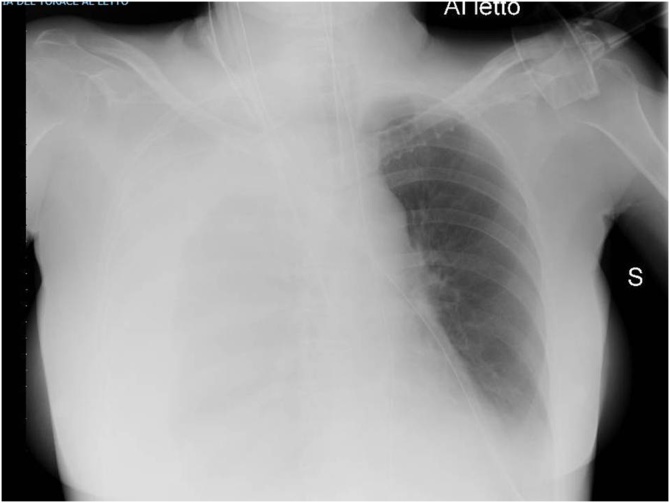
Hemothorax on chest X-ray.
